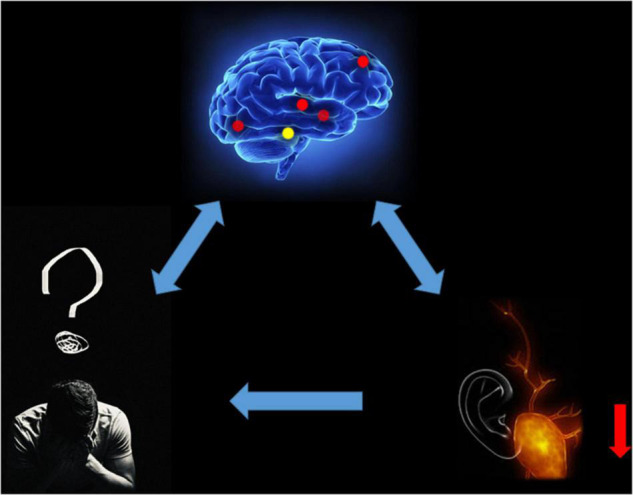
Relationship between MRI images and clinical manifestations in MCI. MCI, mild cognitive impairment.
